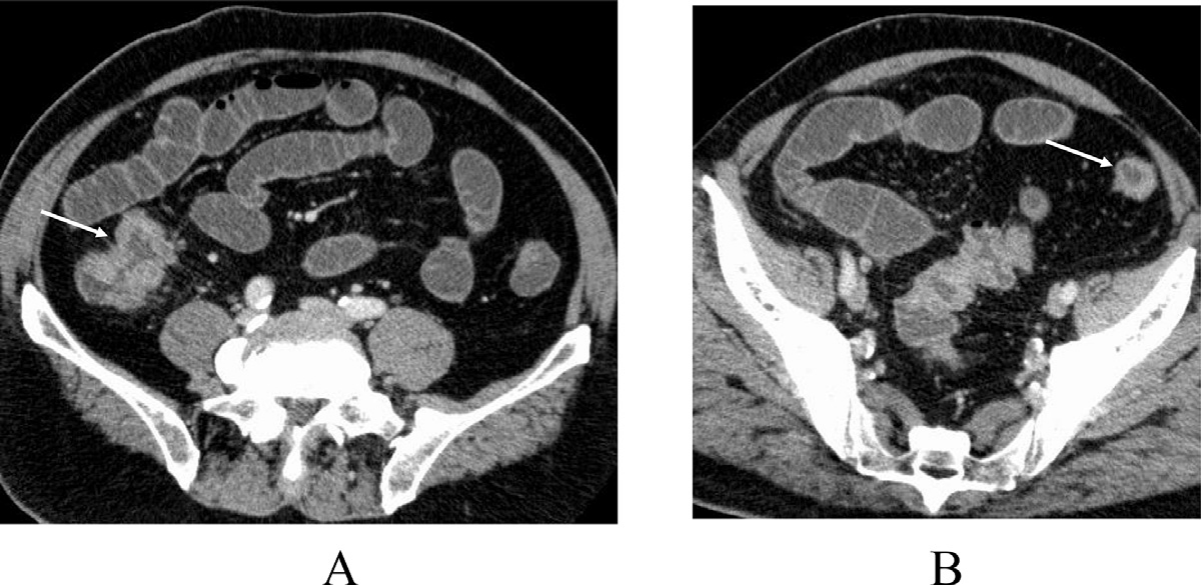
Abdominal computed tomography. (A) An irregular mass (arrow) was observed at the cecum. (B) A small mass with microlobulated boarders (arrow) was observed in the left lower abdomen.
